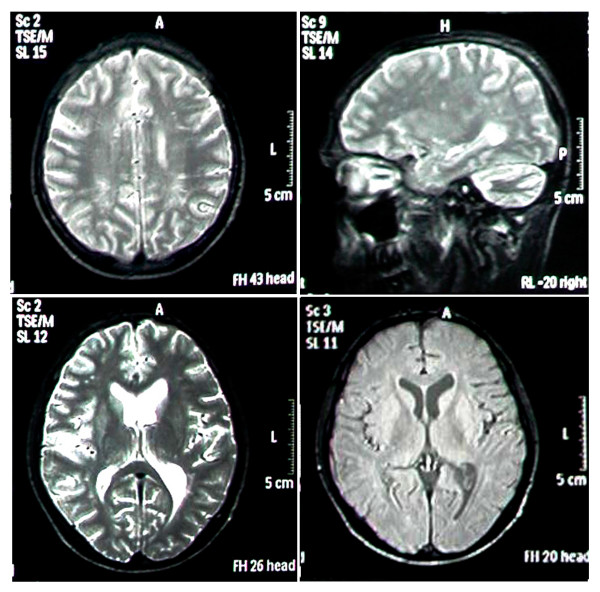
Brain MRI showing white matter changes (hyperintensities) in different parts such as periventricular area, thalamus, and centrum semiovalis.
